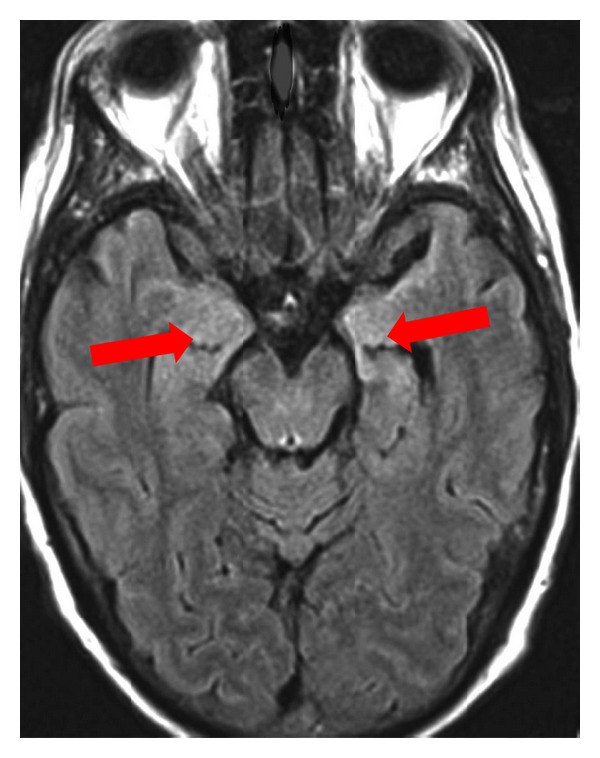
MRI FLAIR of a patient with limbic encephalitis and positive NMDAR antibodies in the CSF. Increased signal intensity is seen in the bilateral medial temporal lobes and hippocampi.
